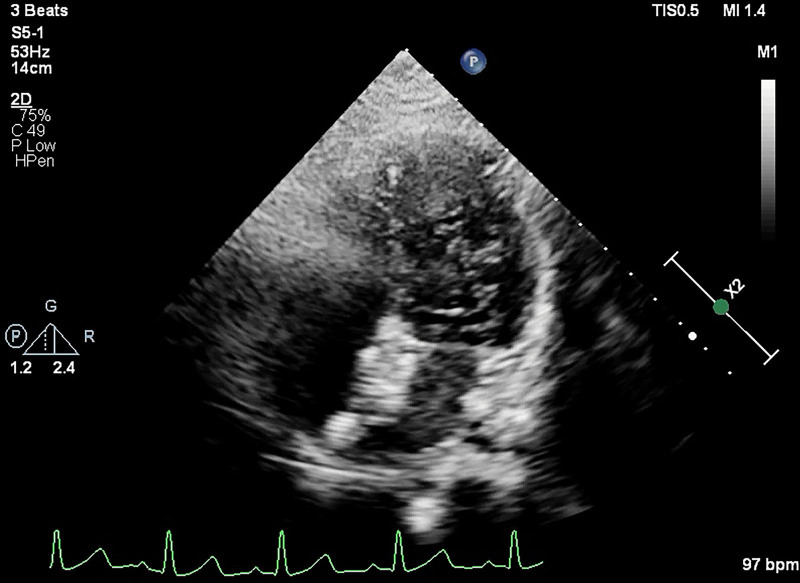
Large number of microbubbles were found at the left-sided heart chambers simultaneously after the effusion of the right-sided heart chambers showed by agitated saline contrast transthoracic echocardiography through the right medial malleolus vein.
